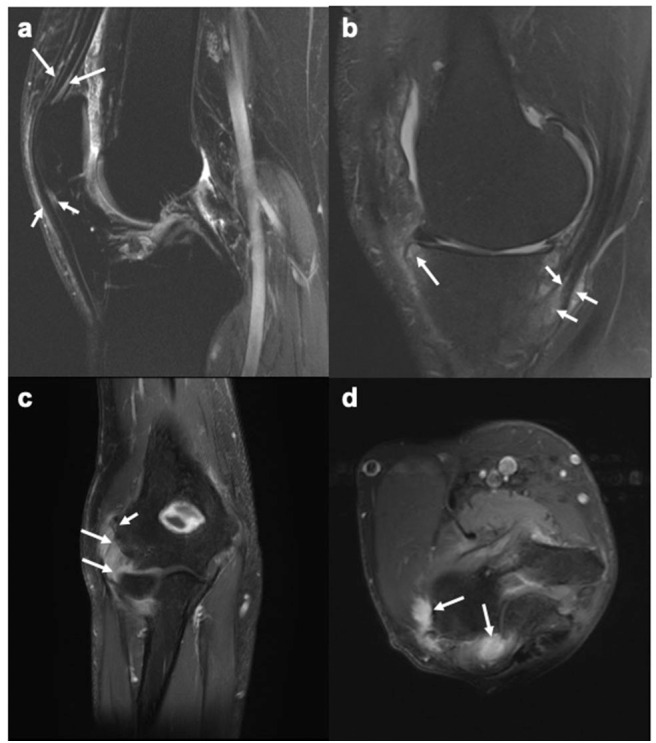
MR images of the knee and elbow depicting enthesitis. (a) Sagittal STIR image of the knee showing soft tissue high signal intensity (intra- and peritendinous) at the insertions of the quadriceps tendon (long arrows) and the patellar ligament (short arrows) at the patella, suggesting enthesitis. (b) Sagittal T2-weighted fat suppressed image of the knee showing high signal intensity (intra- and peritendinous) in the soft tissues of the pes anserine (short arrows), indicating pes anserine enthesitis, as well as bone marrow edema (long arrows) close to the insertion of the medial patellar retinaculum at medial tibial plateau. (c,d) Coronal (c) and axial (d) STIR images of the elbow showing bone marrow edema (mild, short arrow) and soft tissue high signal intensity (long arrows) at the common extensor tendon insertion at the lateral epicondyle, indicating enthesitis. Images courtesy of Professor Iris Eshed, Sackler School of Medicine, Tel Aviv University, Tel Aviv, Israel.
